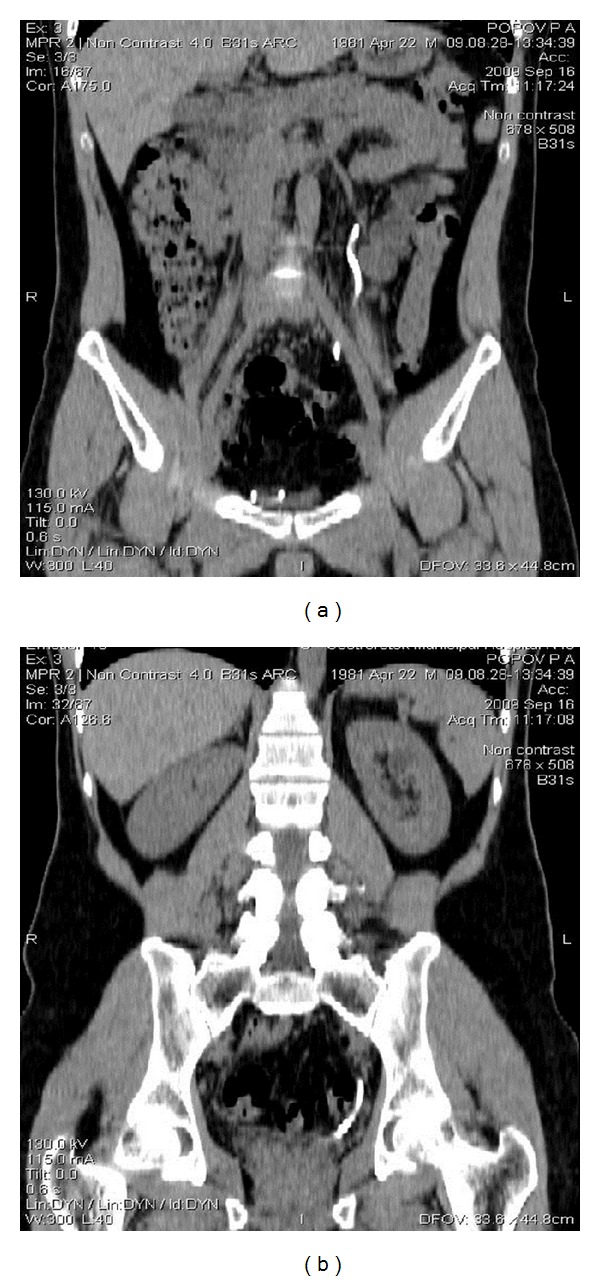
Control CT (3 weeks after stenting and immediately after stent removal)—no extravasation of the contrast medium.
